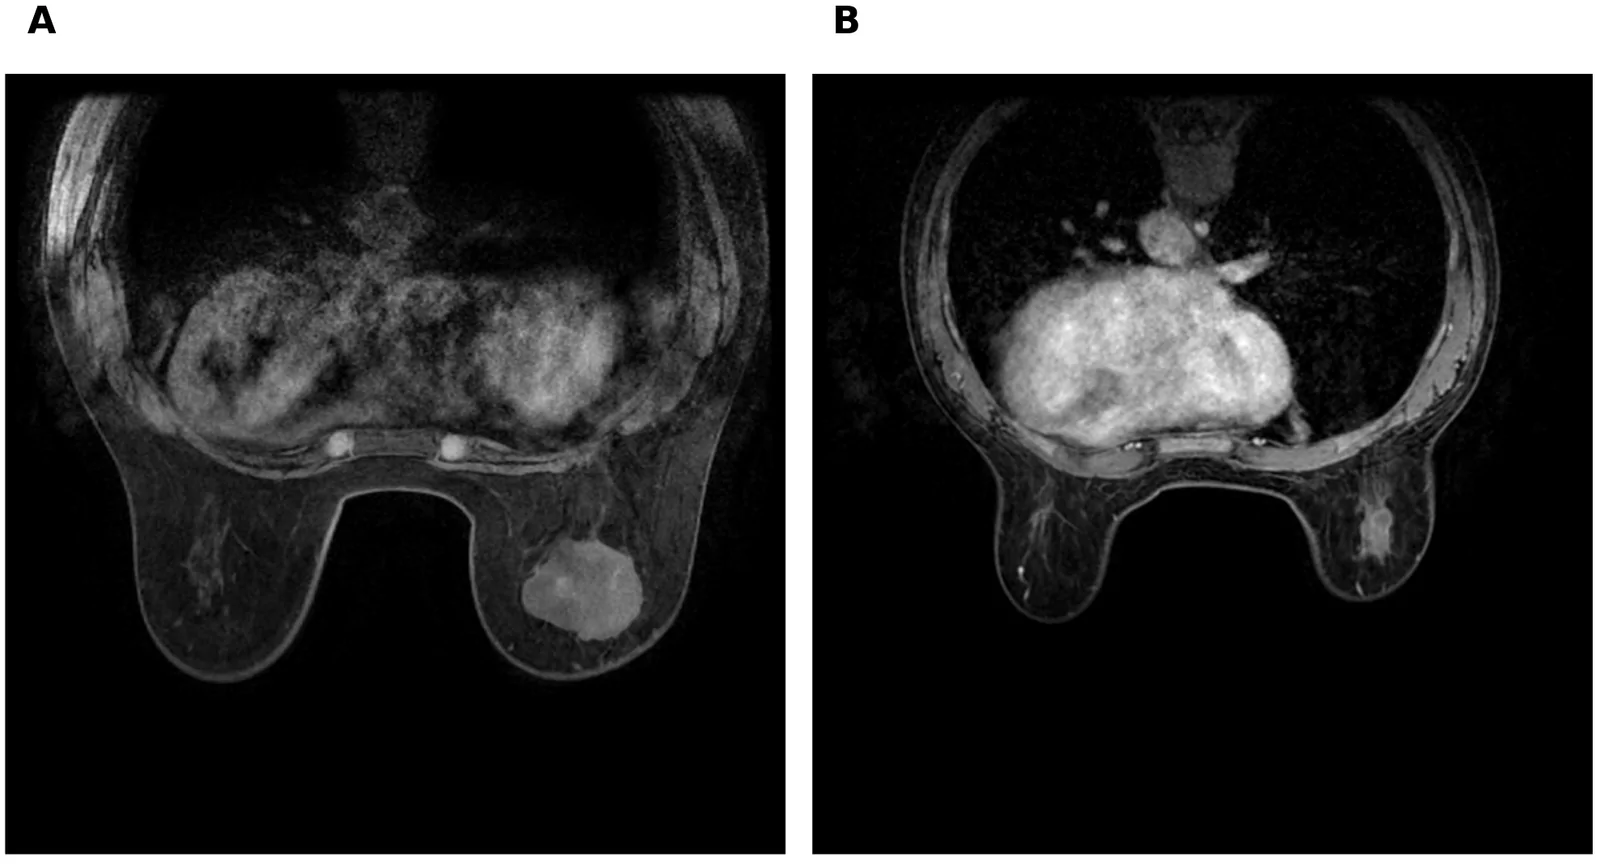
Axial contrast-enhanced breast MRI before and after neoadjuvant therapy. (A) Baseline contrast-enhanced MRI showing an irregular mass measuring approximately 5.1 x 4.1 cm with heterogeneous enhancement. (B) Post-neoadjuvant contrast-enhanced MRI showing marked reduction of the lesion to approximately 2.4 x 1.2 x 1.7 cm and decreased enhancement. .
